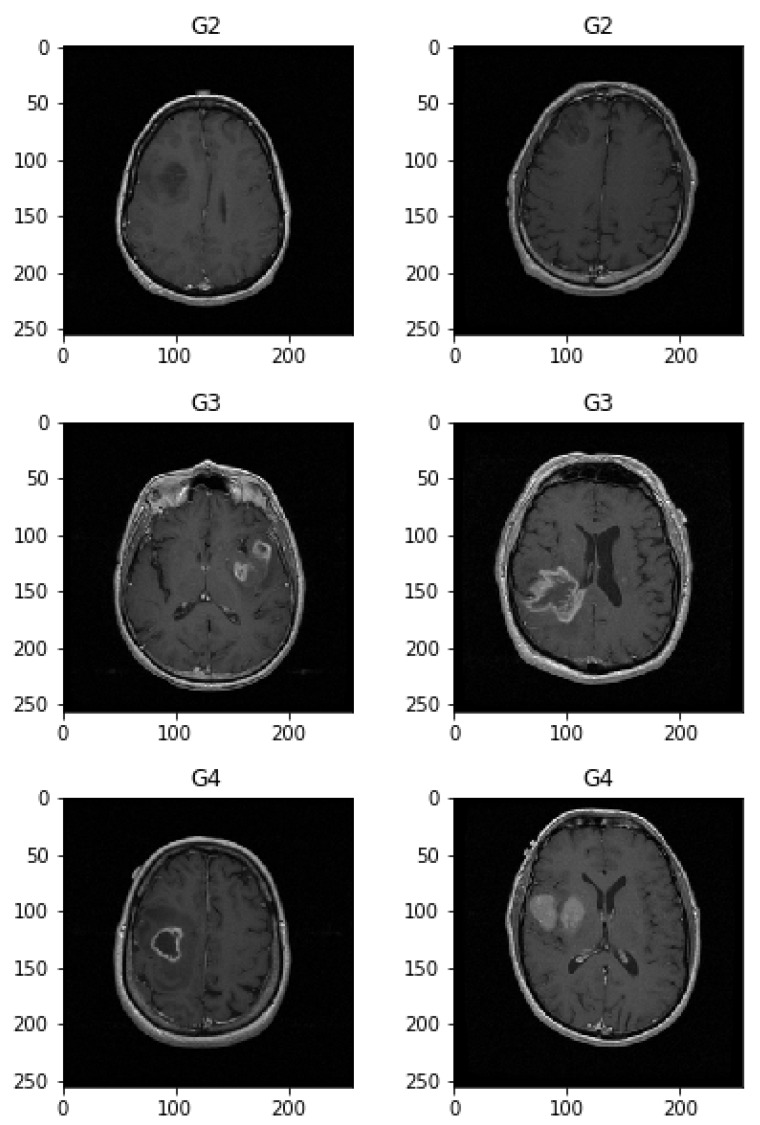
Axial MRI samples of different glioma grades: (top) glioma grade 2; (middle) glioma grade 3; (bottom) glioma grade 4.
